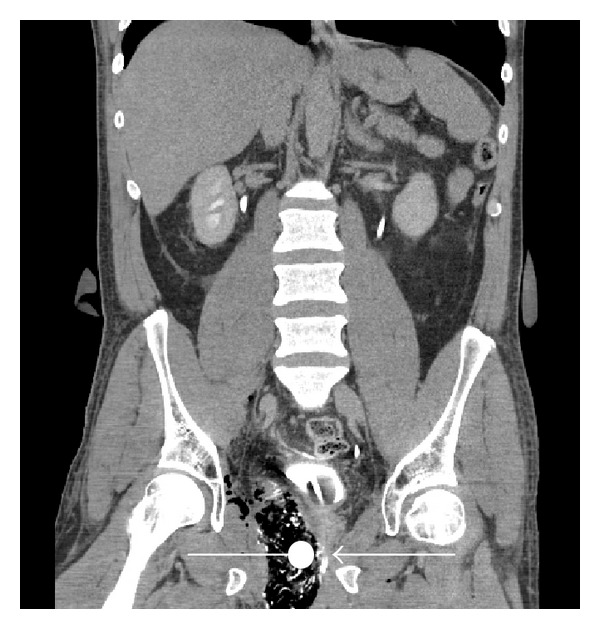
CT scan showing a big swab from the perineum into small pelvis to the right. Note that the swab (round mark) lies directly adjacent to the membranous urethra/transurethral catheter (arrow).
